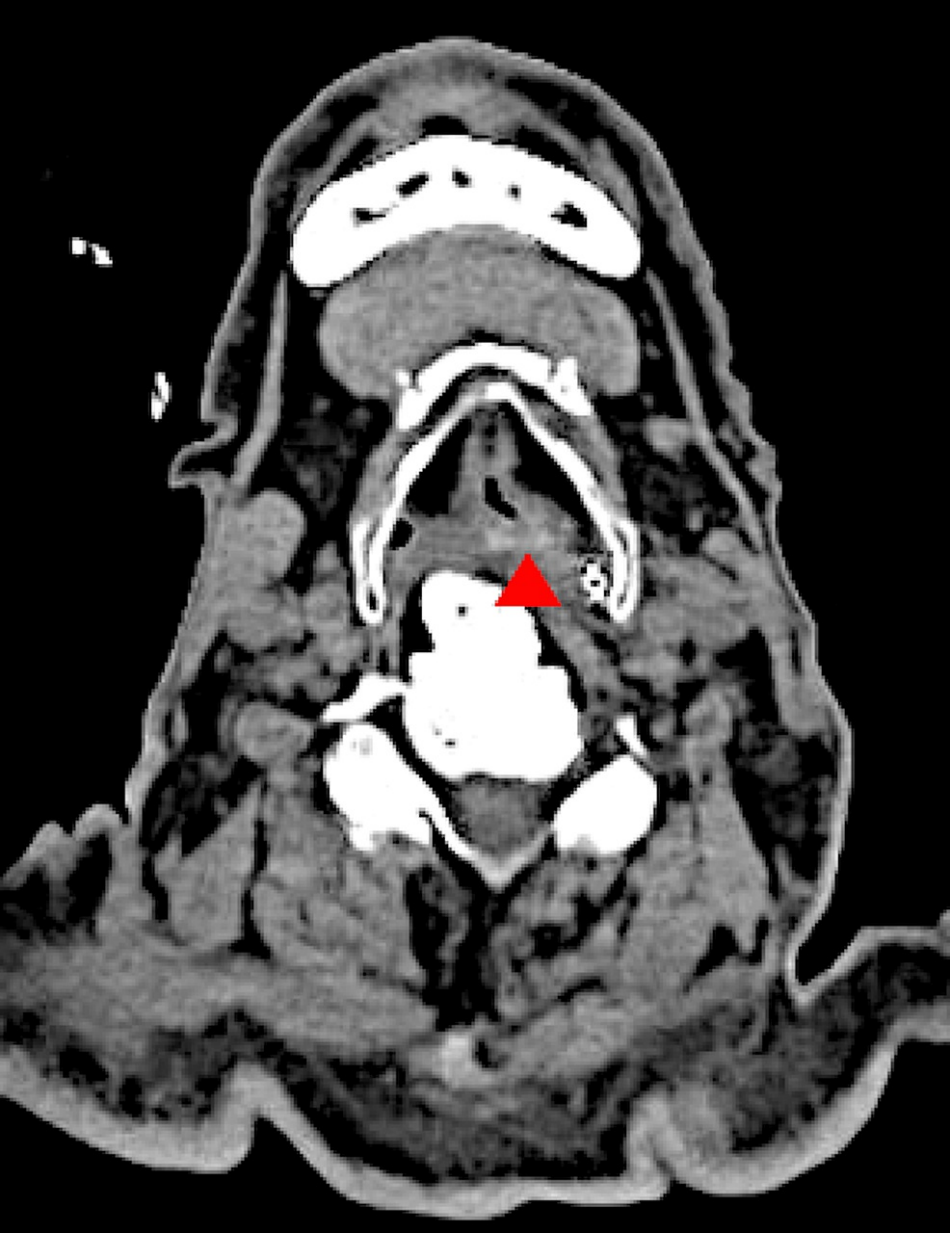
CT scan of the neck Cervical osteophytes (red arrow) compress the tracheoesophageal groove and glottis.
